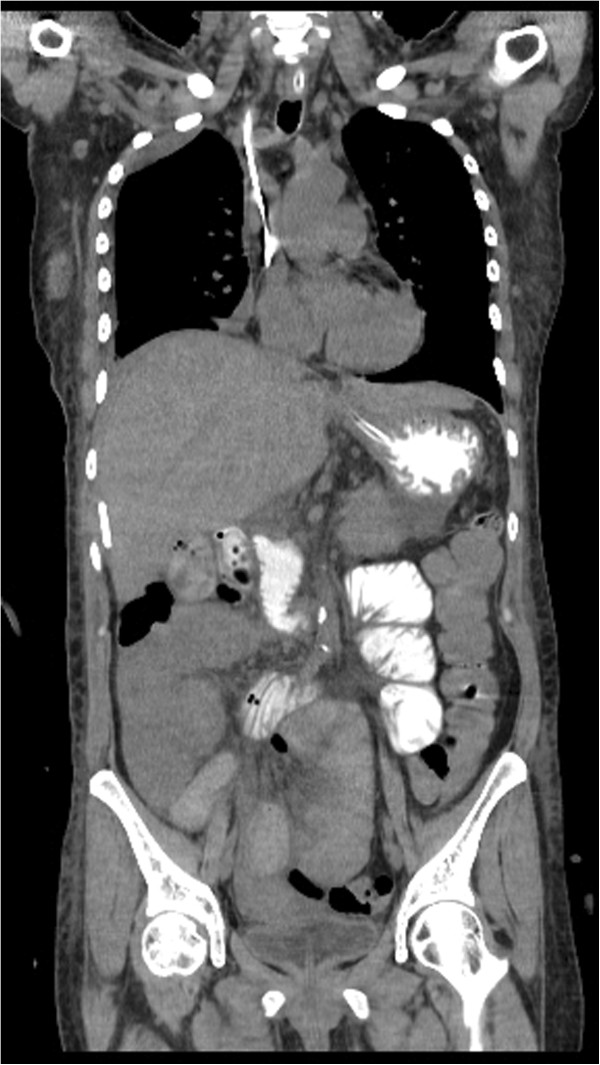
Computed tomography image (coronal view) showing small bowel obstruction associated with a subphrenic collection.
